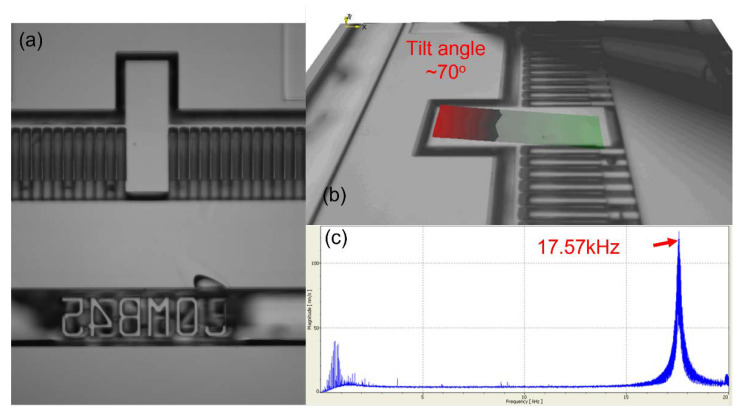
(a) SEM image of device (b) laser Doppler vibrometer results showing maximum tilt angle of 70° using AC bias of 2 V, (c) Fourier transform curve showing resonant frequency at 17.57 kHz.
